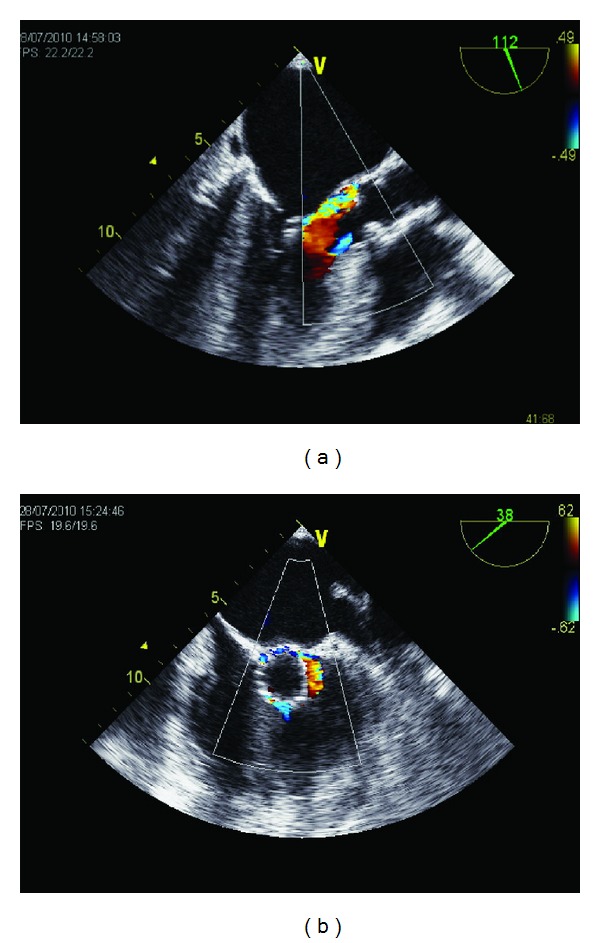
Transesophageal echocardiography images (intercomissural long axis view (a), short axis view (b)) after transcatheter valve implantation demonstrated a moderate paravalvular aortic regurgitation.
